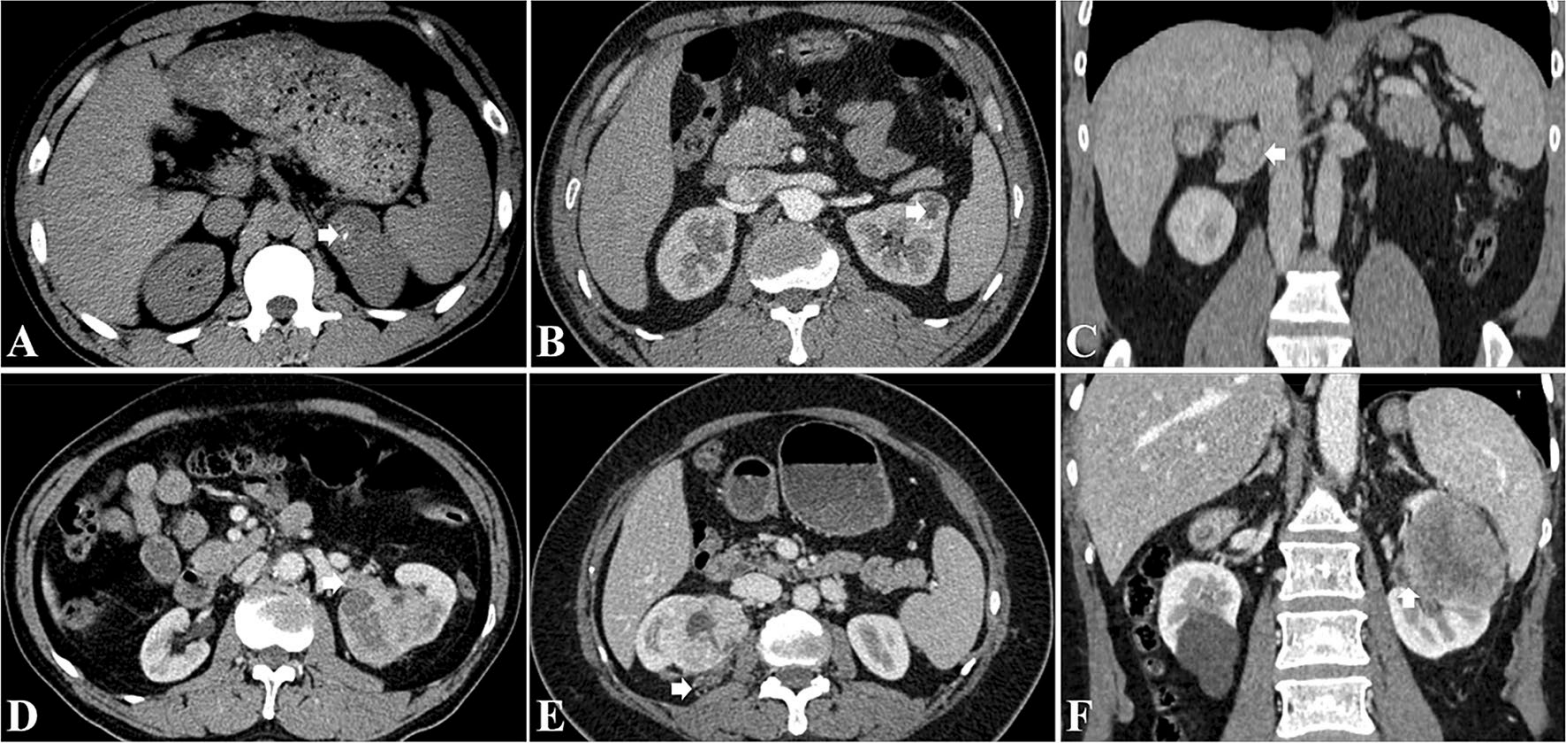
CT imaging features as assessed by both readers: (A) calcification; (B) necrosis; (C) renal vein invasion; (D) renal vein thrombus; (E), perirenal fat invasion; (F) renal sinus invasion.
